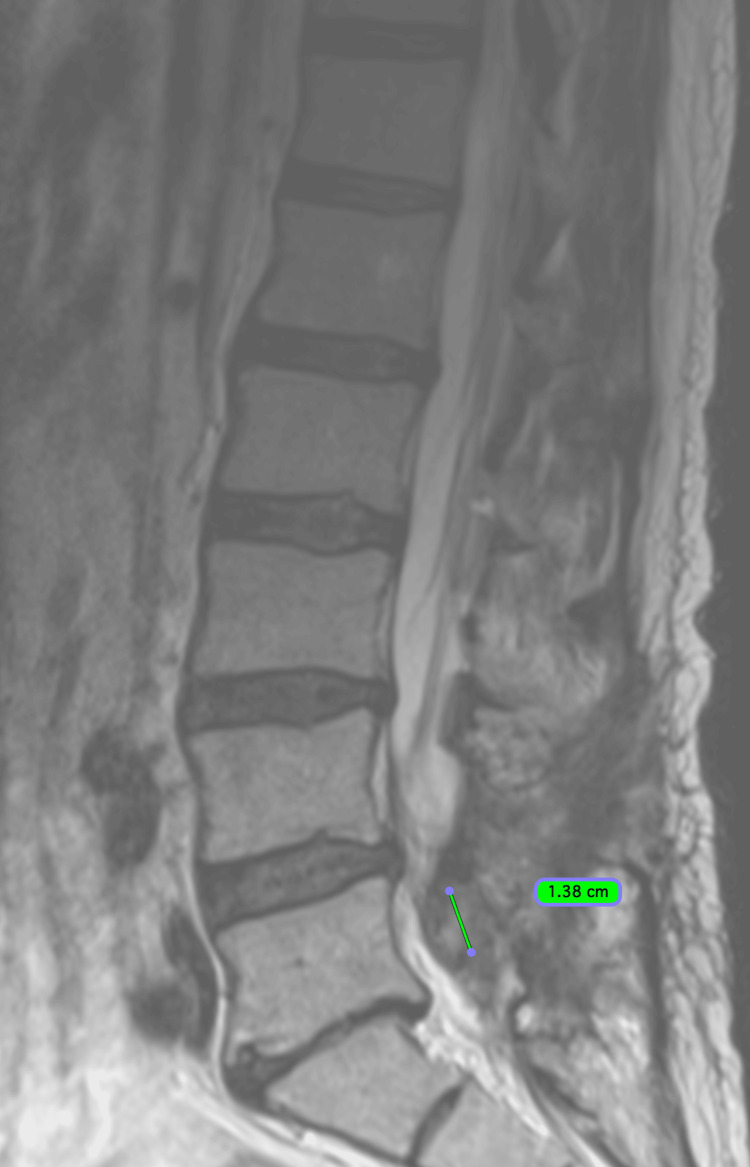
Sagittal image from an MRI L-spine with contrast dated October 10, 2024. Approximately 17 months post cryoablation, the tumor measured 1.38 cm superiorly to inferiorly.
